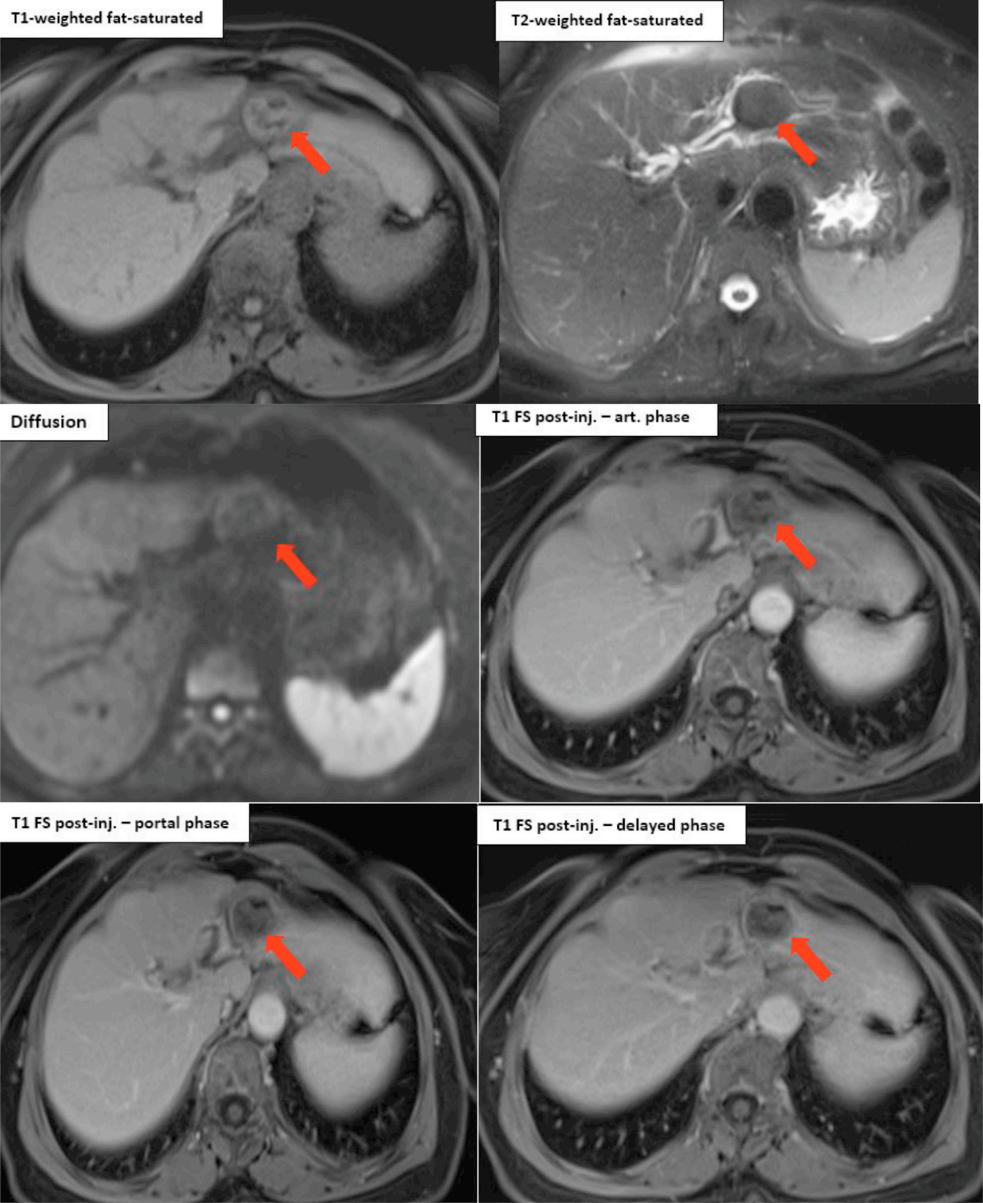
MRI of the liver. Post-segmentectomy changes of segments II and III, with identification of a well-defined, rounded lesion at the resection margin. The lesion appears hypointense on T2-weighted images, isointense on T1-weighted images, contains fatty areas showing signal drop on Dixon in-phase/out-of-phase sequences, and demonstrates moderate peripheral enhancement after contrast administration (red arrow).
